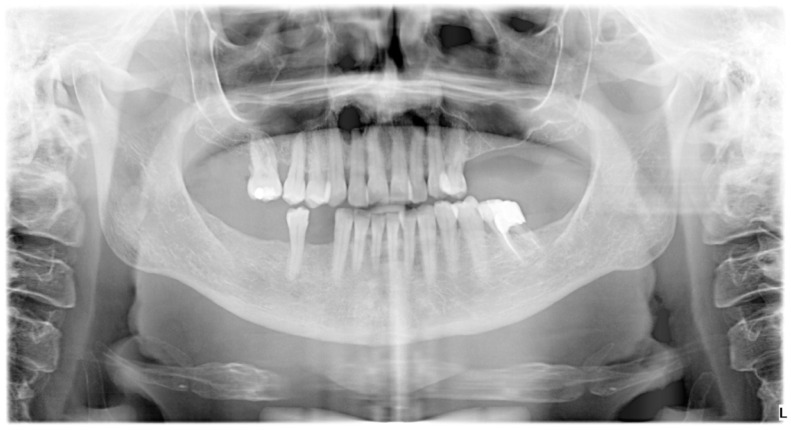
Orthopantomogram (OPG) showing a solitary, hollow area that appears dark on an X-ray image, in the front upper jaw on the right side, specifically around the base of the upper right lateral incisor.
